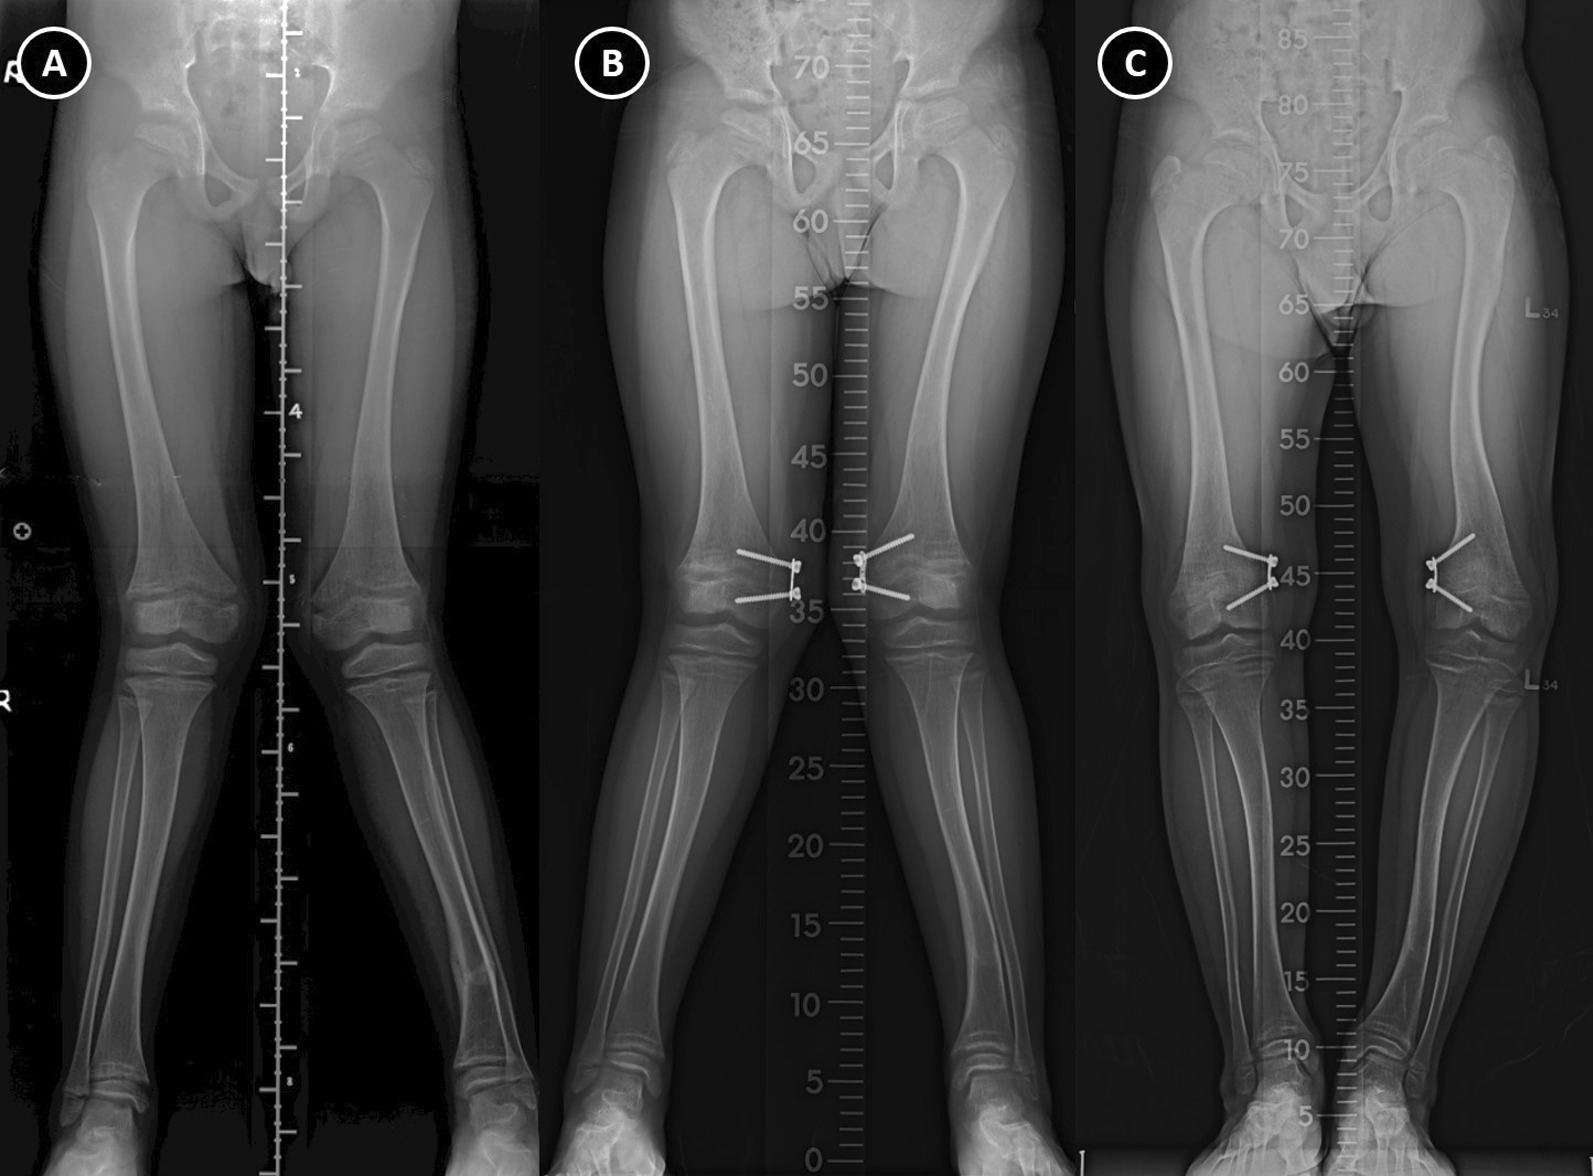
A 9-year-old male with hypophosphatemic rickets presented with bilateral genu valgum (A). Growth modulation performed at 10.2 years at bilateral distal femur (B). Latest radiographs before implant removal demonstrating overcorrection into varus bilaterally (C)
